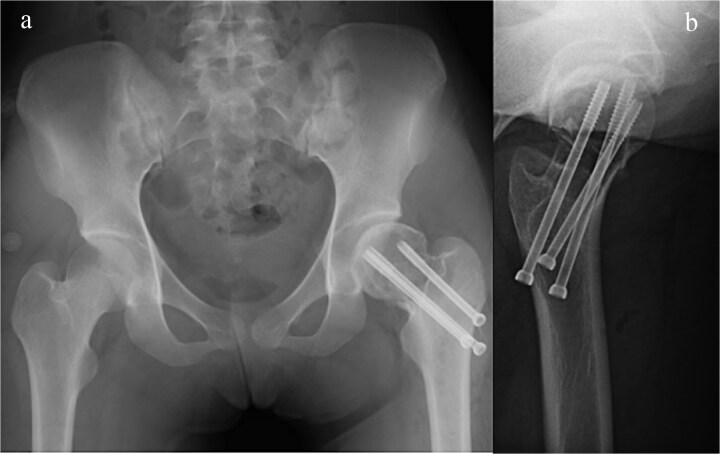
Plain radiograph after initial surgery. A fracture site was stabilized by three canulated screws, there was significant bone defect in the femoral head and neck. (a) A-P view and (b) lateral view.
